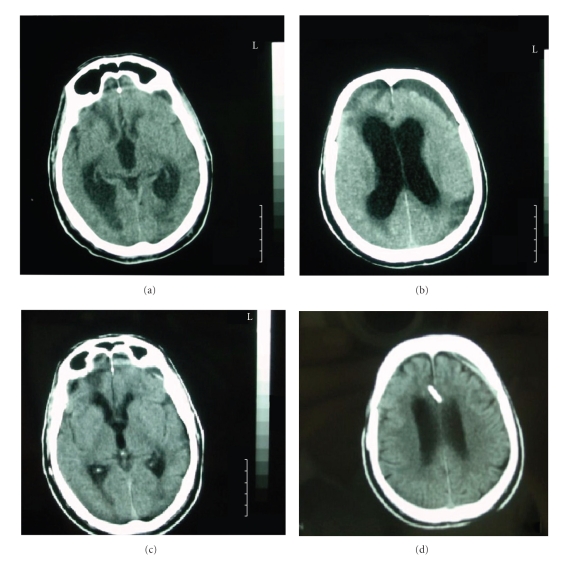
CT scan showing the left frontal subdural effusion associated with hydrocephalus (a) and (b) treated with V-P shunt.
